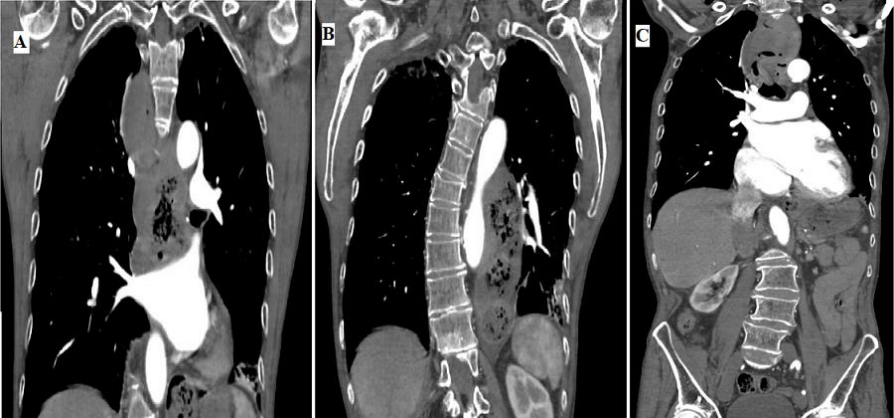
A) thoracic-abdominal computed tomography (CT) scan demonstrated mega-esophagus with an airfluid levels; B) thoracic-abdominal CT scan demonstrated a dilated and tortuous esophageal lumen; C) thoracic-abdominal CT scan demonstrated numerous food ingestis
